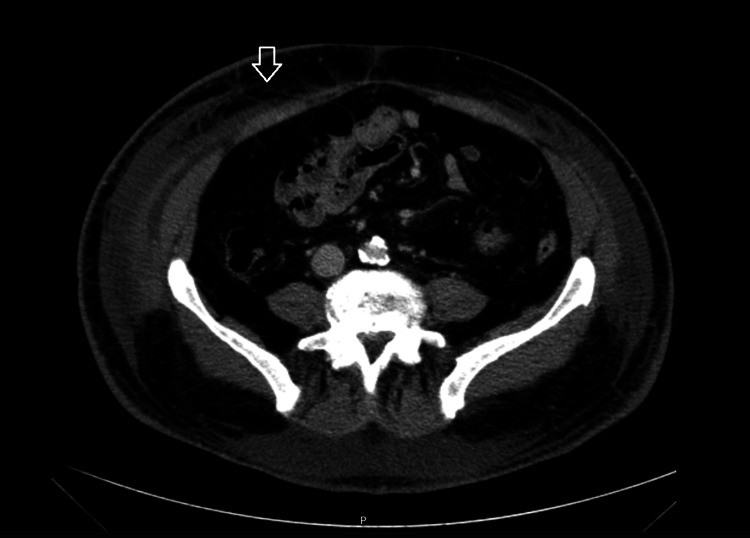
Thoracoabdominal CT scan of patient 4
